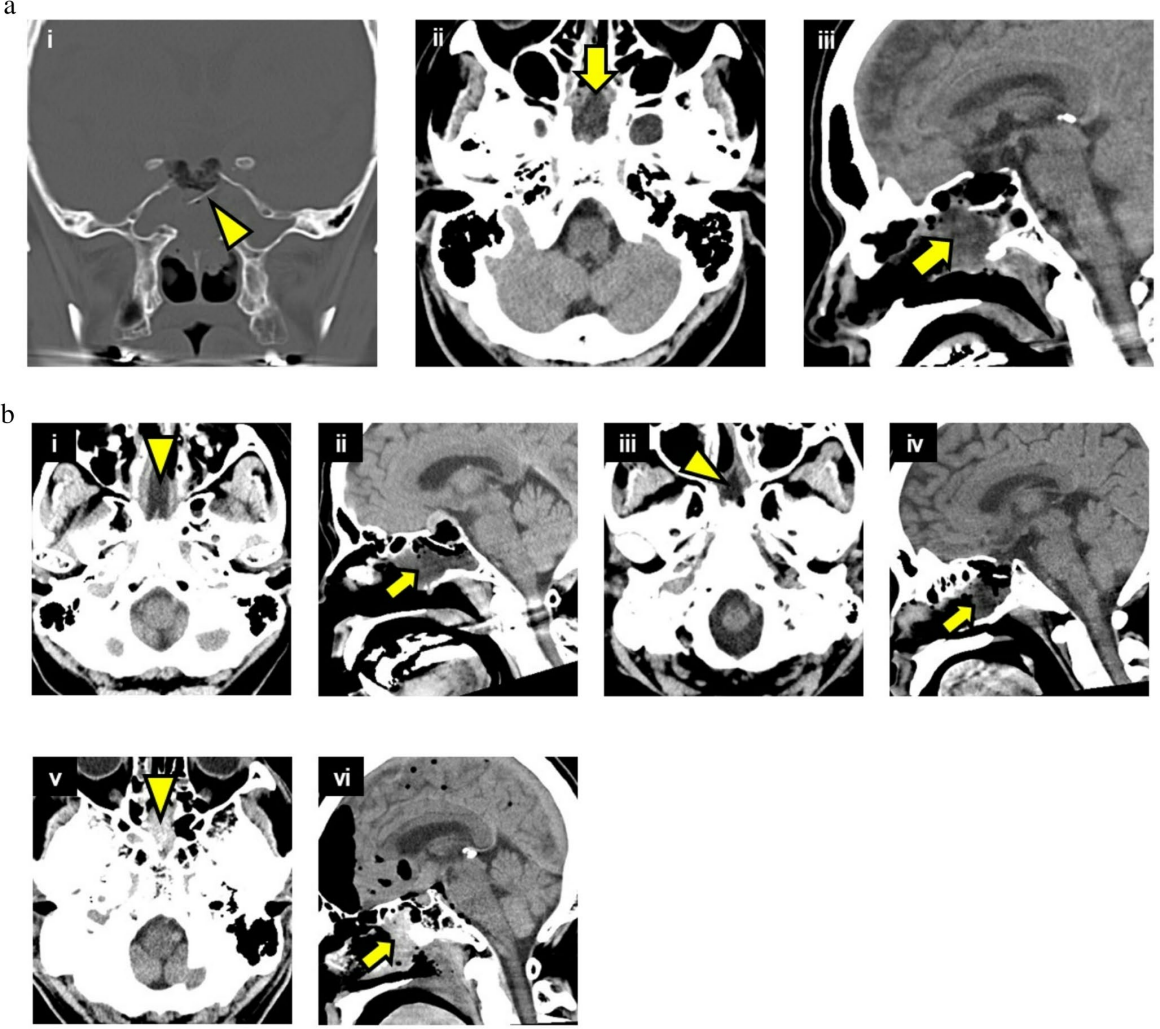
Postoperative computed tomography (CT) findings in Case 10 and early cerebrospinal fluid leakage sign a: Case 10: A 57-year old man. Postoperative CT revealed prolapse of the nasal septal bone (arrowhead in ⅰ) and cerebrospinal fluid retention under the nasal mucosa and within the sphenoid sinus (arrows in ⅱ and ⅲ). b: Early cerebrospinal fluid leakage sign
